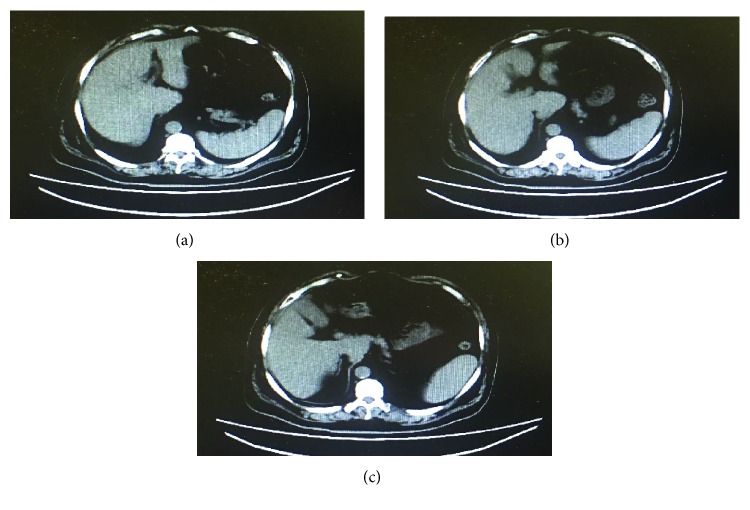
(a–c) Abdominal CT of one patient with the low fecal elastase level (less than 100 *μ*g/g) showed atrophy of the pancreas.
